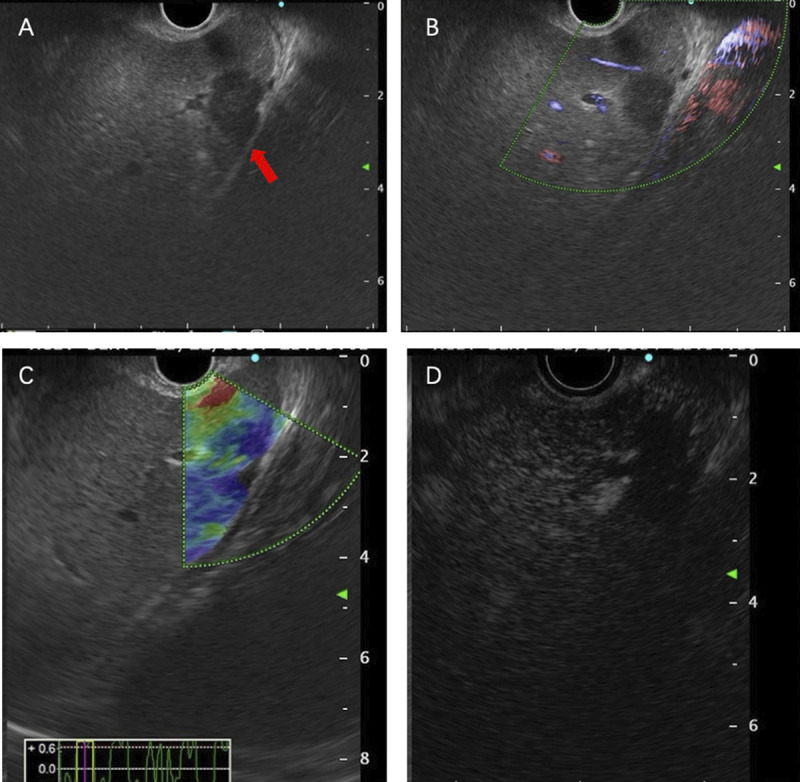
EUS (A) showed a quasi-circular hypoechoic lesion in the left lobe of the liver, approximately 15 mm × 12 mm. Color Doppler flow imaging (B) showed no significant blood flow signals. Elastography (C) displayed blue-green coloration. Contrast-enhanced EUS imaging (D) revealed weak enhancement.
